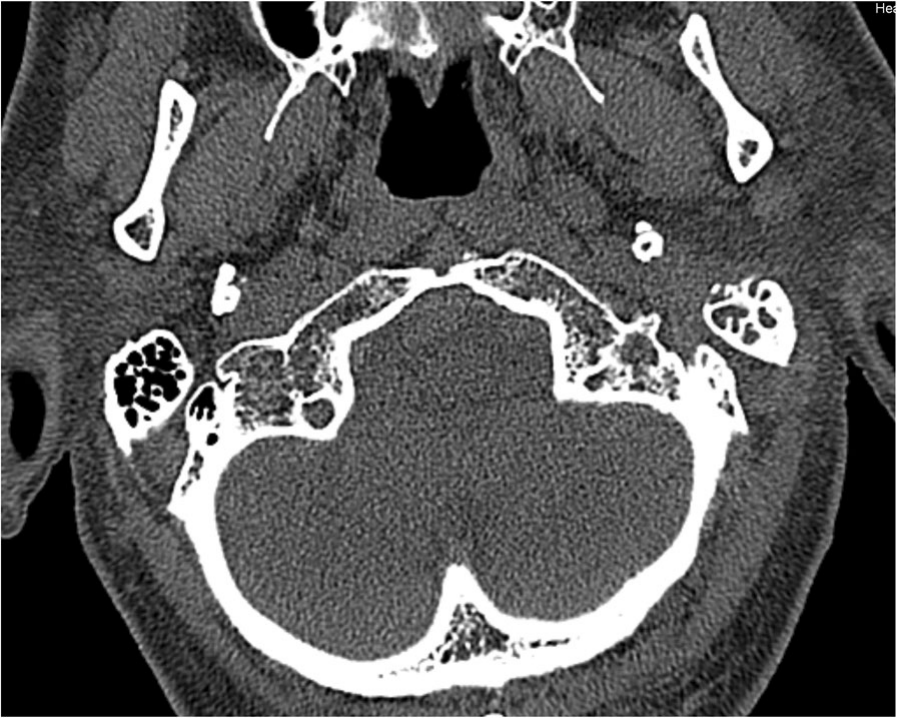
Day 0. Non-contrast enhanced CT in axial plane, section thickness 1 mm. The fatty tissues adjacent to the stylomastoid foramen are infiltrated, and cortical bone within the mastoid process is discretely eroded. The mastoid cells are filled with oedematous mucosa and soft tissue
